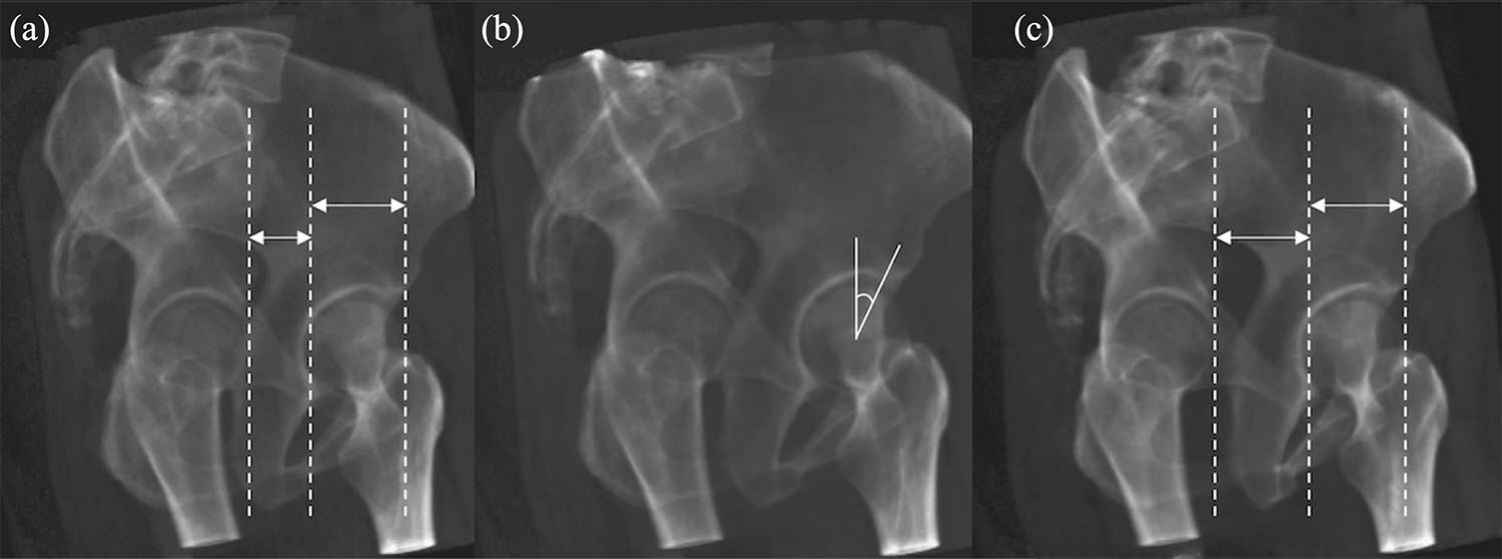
FP radiographs of different pelvic rotations are shown in (a–c). ACEA is measured in a FP radiograph at 65°of rotation (b). Investigated the “appropriate range of positioning” where the distance-to-diameter ratio is between 0.67 (a: 68.1°of rotation) and 1.0 (c: 63.2°of rotation). The arrows indicate the distance between the femoral heads and the femoral head diameter. *ACEA* Anterior center edge angle, *FP* False profile.
